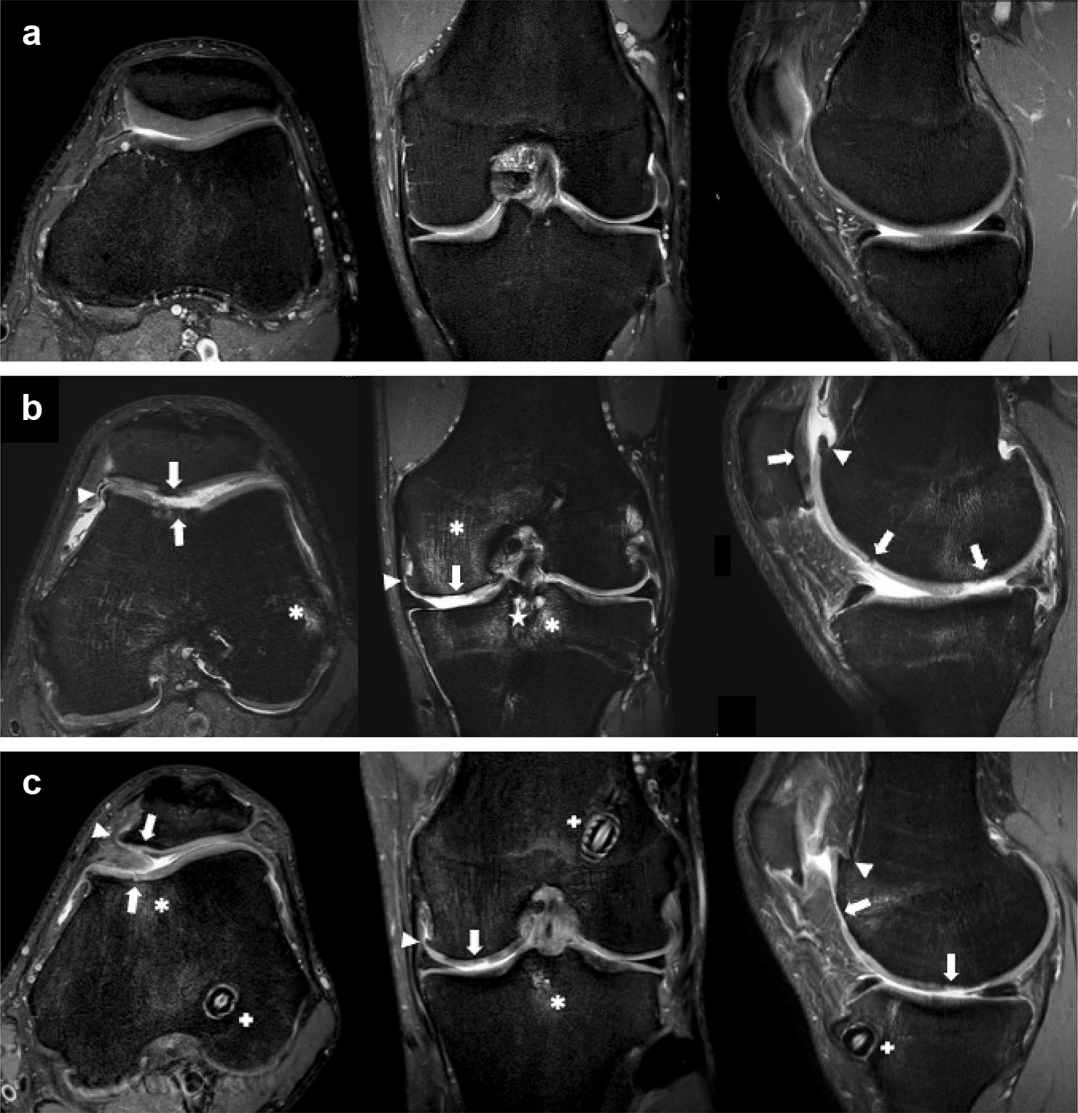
MRI of three soccer players (no prior injuries, prior injuries, no reported prior injuries). a Left knee of a 19-year-old striker with no prior injuries. The WORMS was 2 for both knees; hence, the total WORMS was 2. b Left knee of a 29-year-old midfielder with documented prior injuries. Note the severe cartilage damage, osteophytes and bone marrow oedema. The WORMS was 111 for the left knee and 10 for the right knee; hence, the total WORMS was 61. c Left knee of a 23-year-old defender with no documented prior injuries. The MRI demonstrates advanced joint damage and prior ACL repair. The WORMS was 53 on the left side and 27 on the right. The total WORMS was 40. Cartilage damage: arrows, bone marrow oedema: asterisk, osteophytes: arrowheads, cysts: star, ACL repair: plus sign
